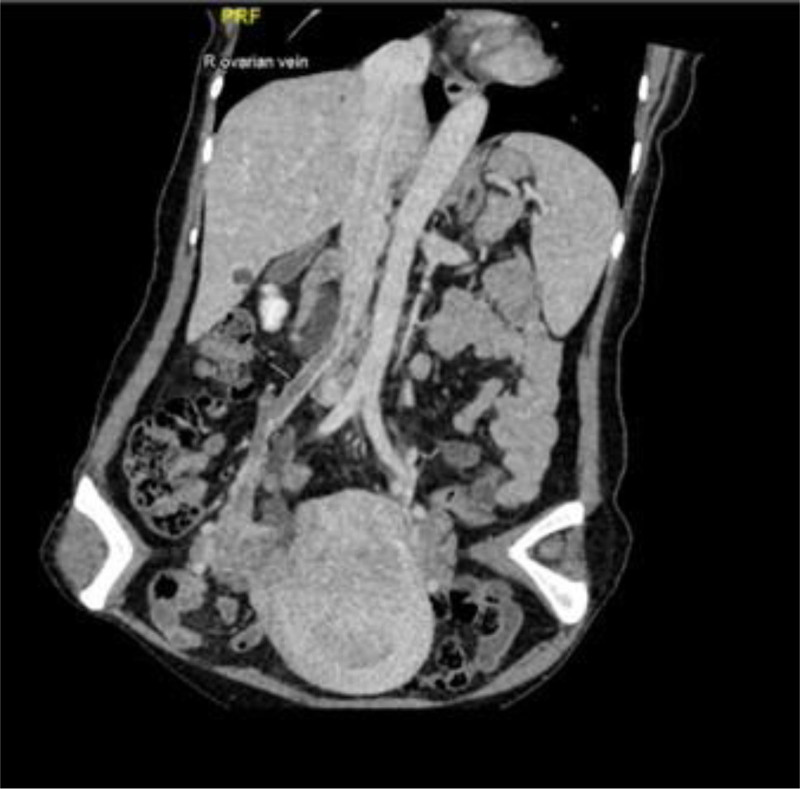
Lower limb deep venous CTV of case 1. CTV showed a mass located in pelvis, extending from the right internal iliac vein to the right atrium, supporting IVL. CTV = computed tomography venography, IVL = intravenous leiomyomatosis.
